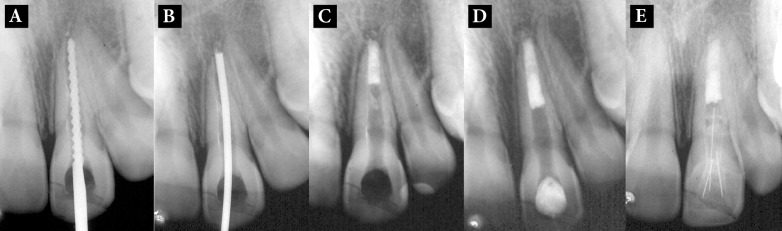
A*) Operative radiography of the maxillary left central incisor with a #140 K-file; *B)* MTA-specific points inside the canal; *C) *placement of the MTA apical plug; *D)* Canal backfilling *E)* Six years later
